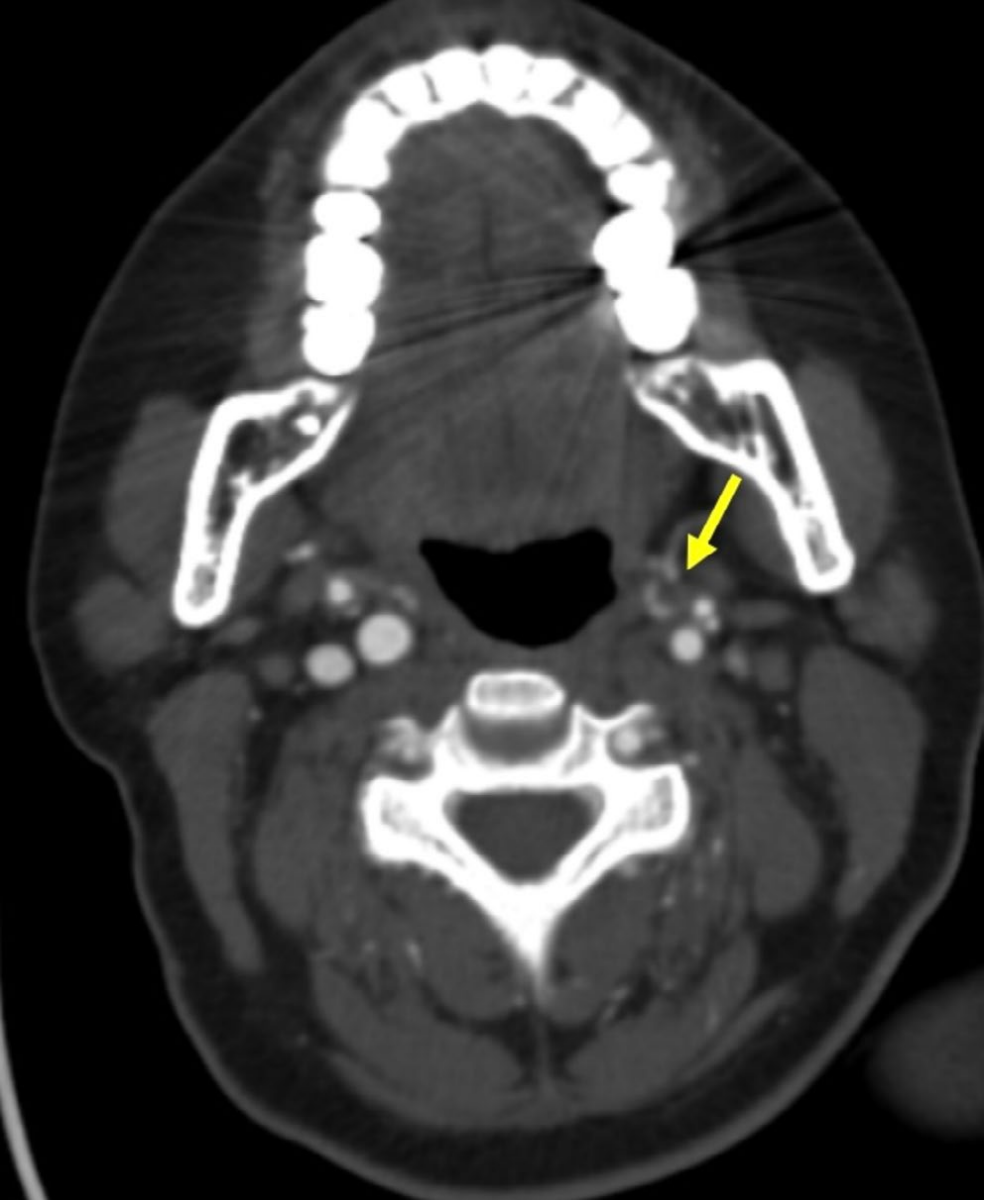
CT angiogram of the head shows nonopacification of the left proximal internal jugular vein (arrow), consistent with thrombotic occlusion.
